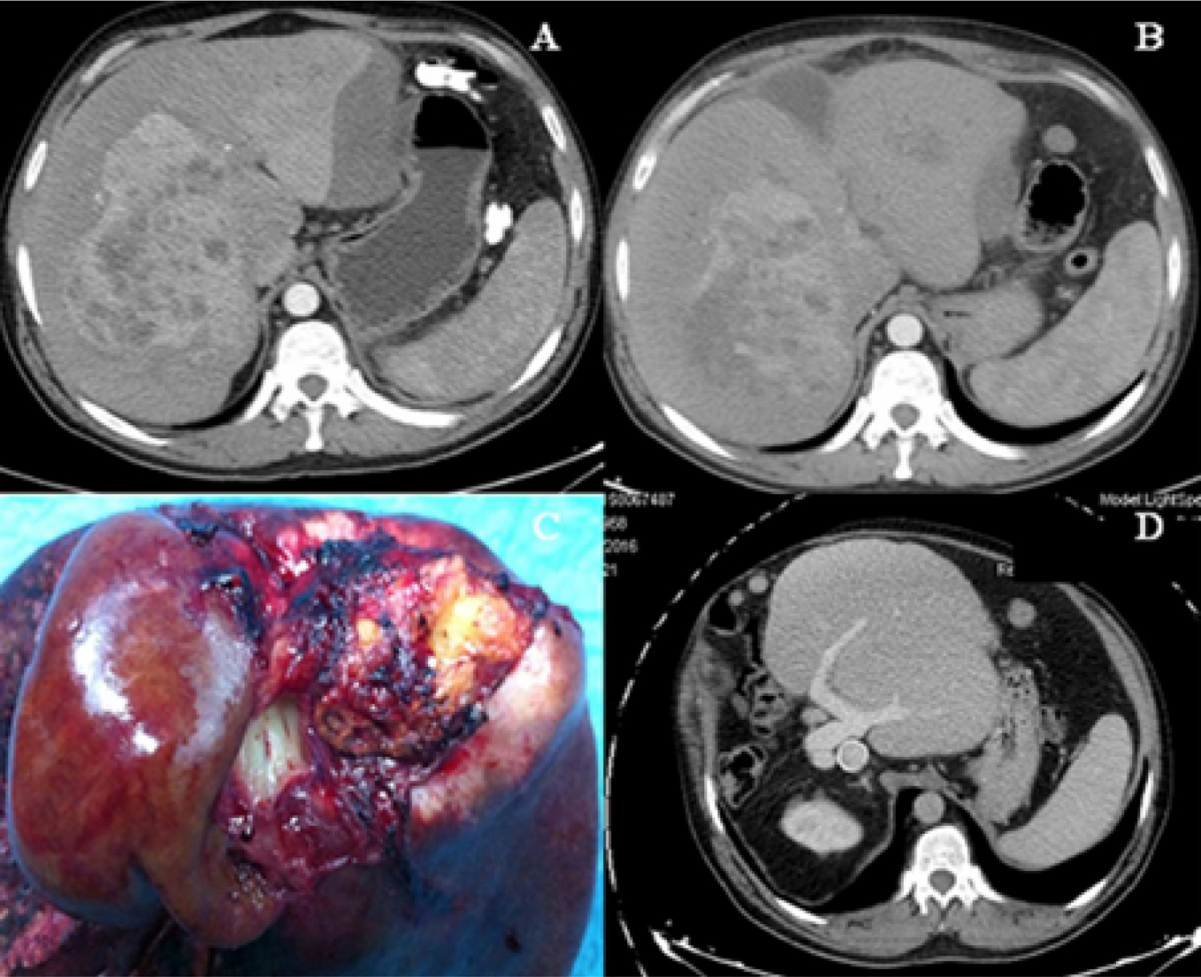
(A) Very large HCC with grade 2/6 fibrosis beyond the BCLC classification and inferior vena cava invasion; cirrhosis was ruled out, but subcapsular hematoma in the left lobe was detected. (B) CT volumetry on day 7 after stage I, with a future liver remnant increase of 187%. (C) Right trisectionectomy with inferior vena cava resection involving the caudate. (D) Follow-up CT at 60 months shows a disease-free liver with the vena cava graft still permeable.
